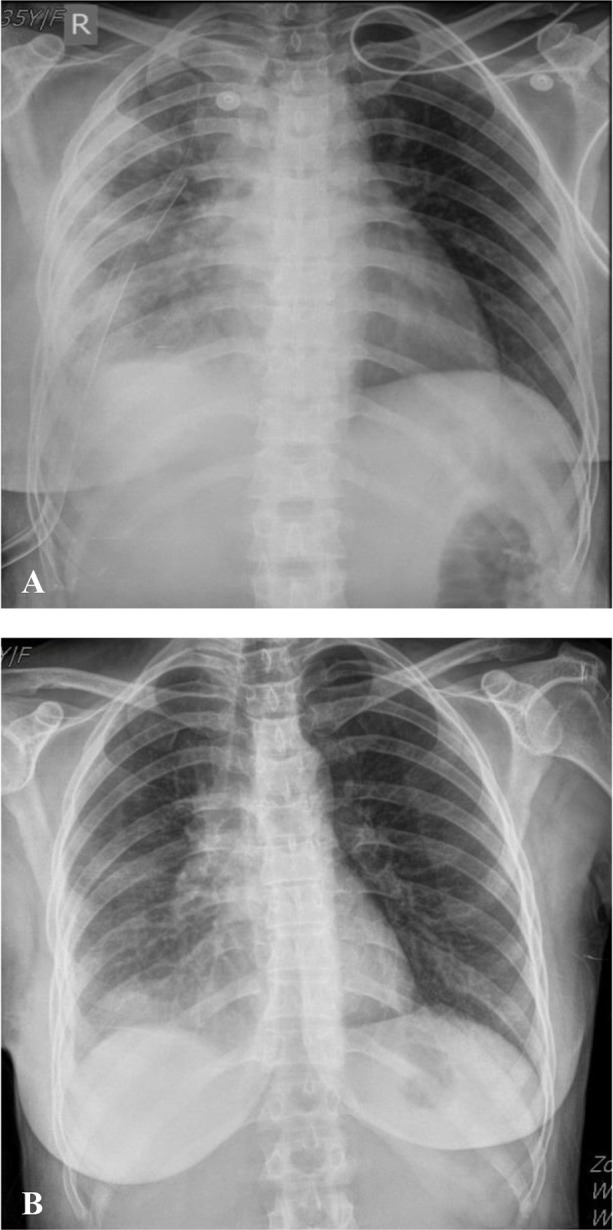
(A) Post-operation chest X-ray radiograph showed expanded right side lungs mild pleural effusion and thoracostomy in it .(B) After one month follow up, chest X-ray radiograph showed a normal pattern.
